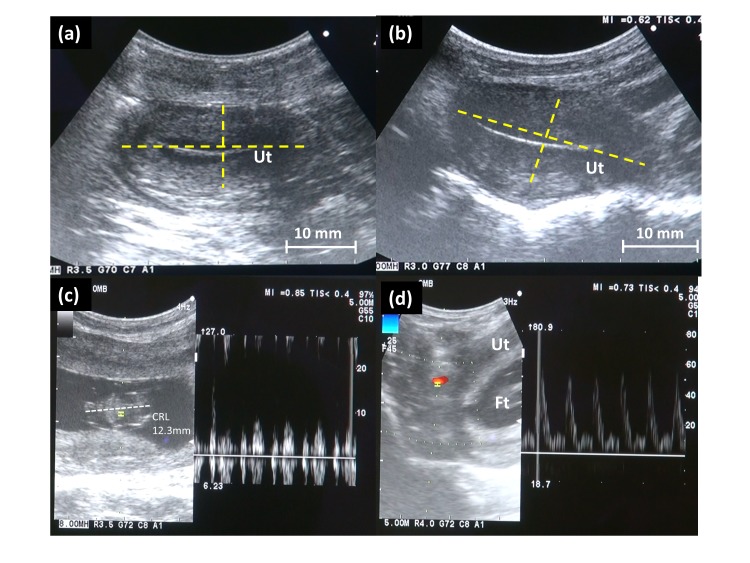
Postoperative evaluation of the uterus. (a) Transabdominal ultrasonography at the time of surgery showed the size of the uterine corpus was 25.2 × 13.1 mm (long axis × anteroposterior diameter) (b) The size of uterine corpus at 2 months after surgery was 25.0 × 13.8 mm, showing no major change in the size of the uterus. Ut: uterus. (c) At 37 days after mating, transabdominal ultrasonography revealed a viable fetus with crown-rump length 12.3mm and regular fetal heart beat. (d) The right uterine artery flow using Doppler ultrasonography was satisfactory and no collateral vessels toward the uterus were shown. Ut: uterus, Ft: fetus.
